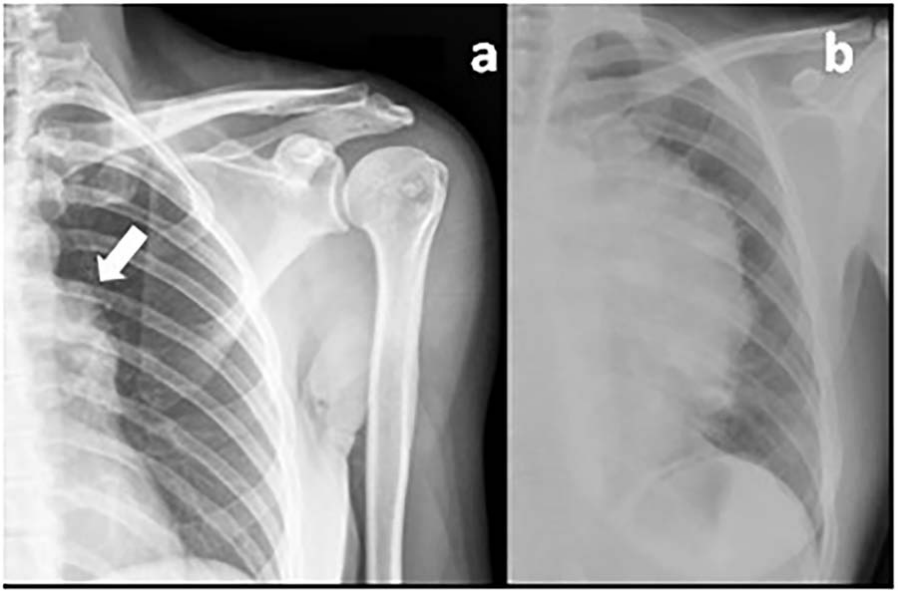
Previous shoulder X-ray (a) showing an irregular shape of the aortic arch (arrow) probably related to the mediastinal mass in comparison with a recent chest X-ray (b) showing the giant thymoma.
